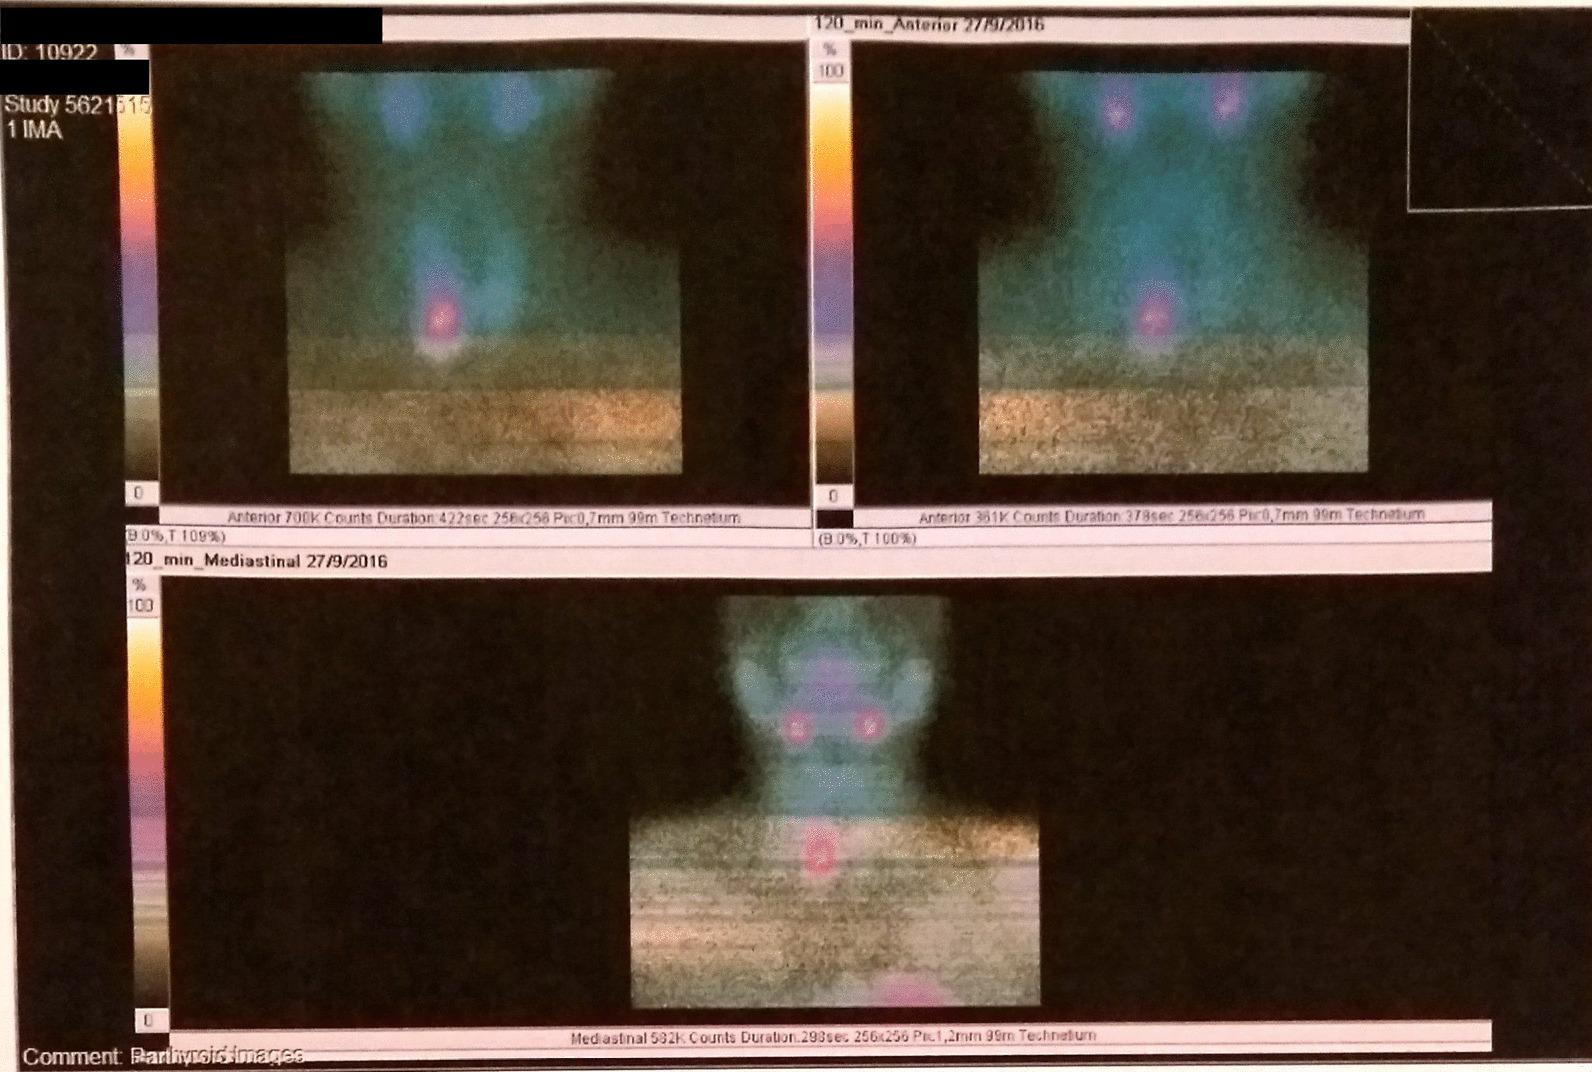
99mTc–Sestamibi scintigraphy revealing an inferior right-sided parathyroid adenoma
